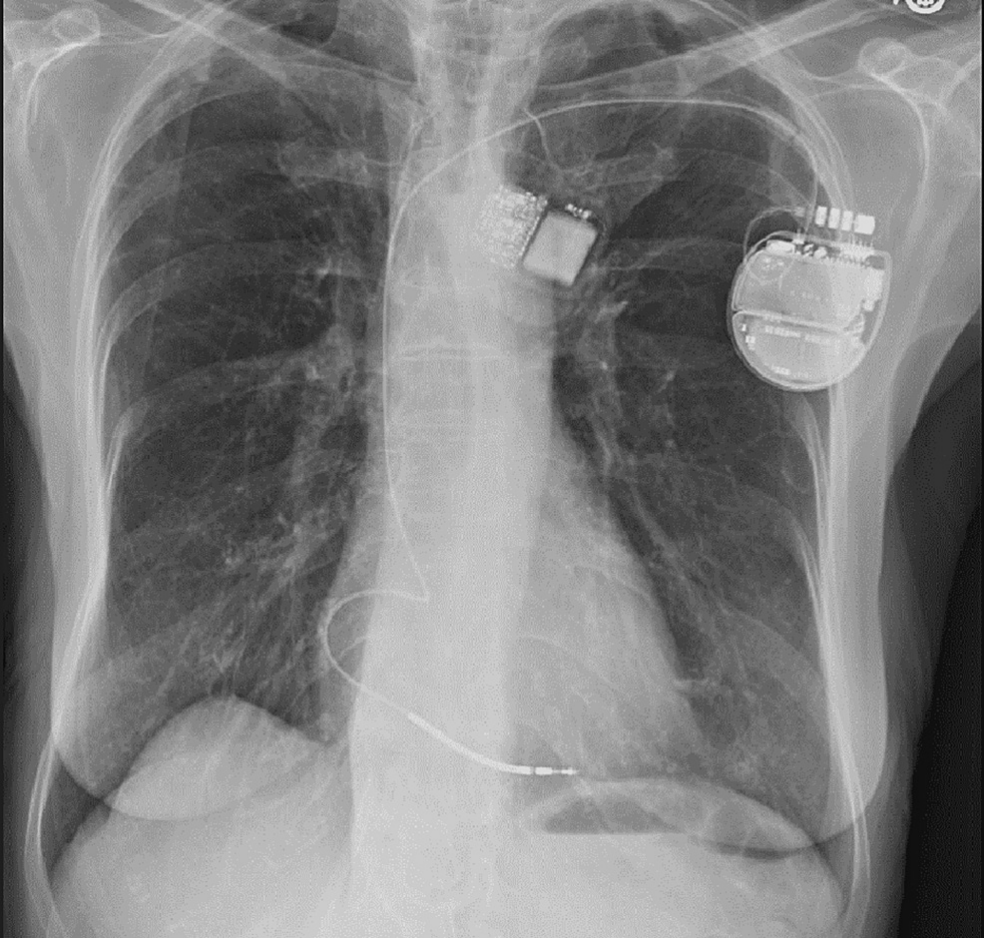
Chest radiograph The radiograph indicates the location of the ICD generator
